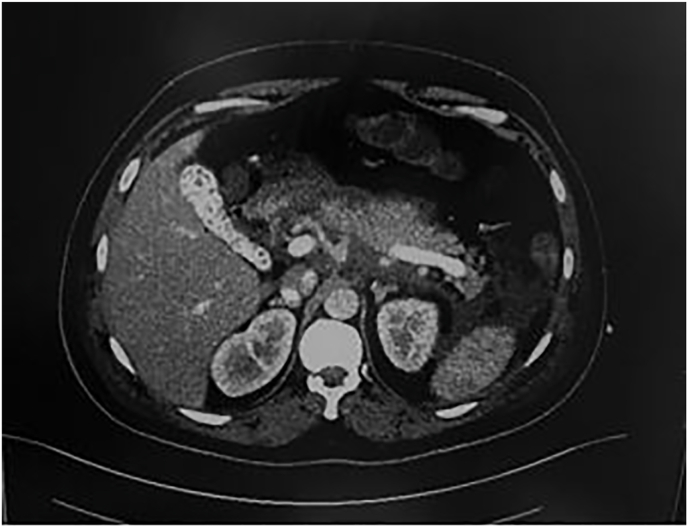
The computed tomography findings showed severe AP with extensive inflammatory stranding around the pancreas, free fluid in the left paracolic gutters (grade E of Balthazar classification), and a gallbladder stone with no evidence of biliary dilatation or free air.
